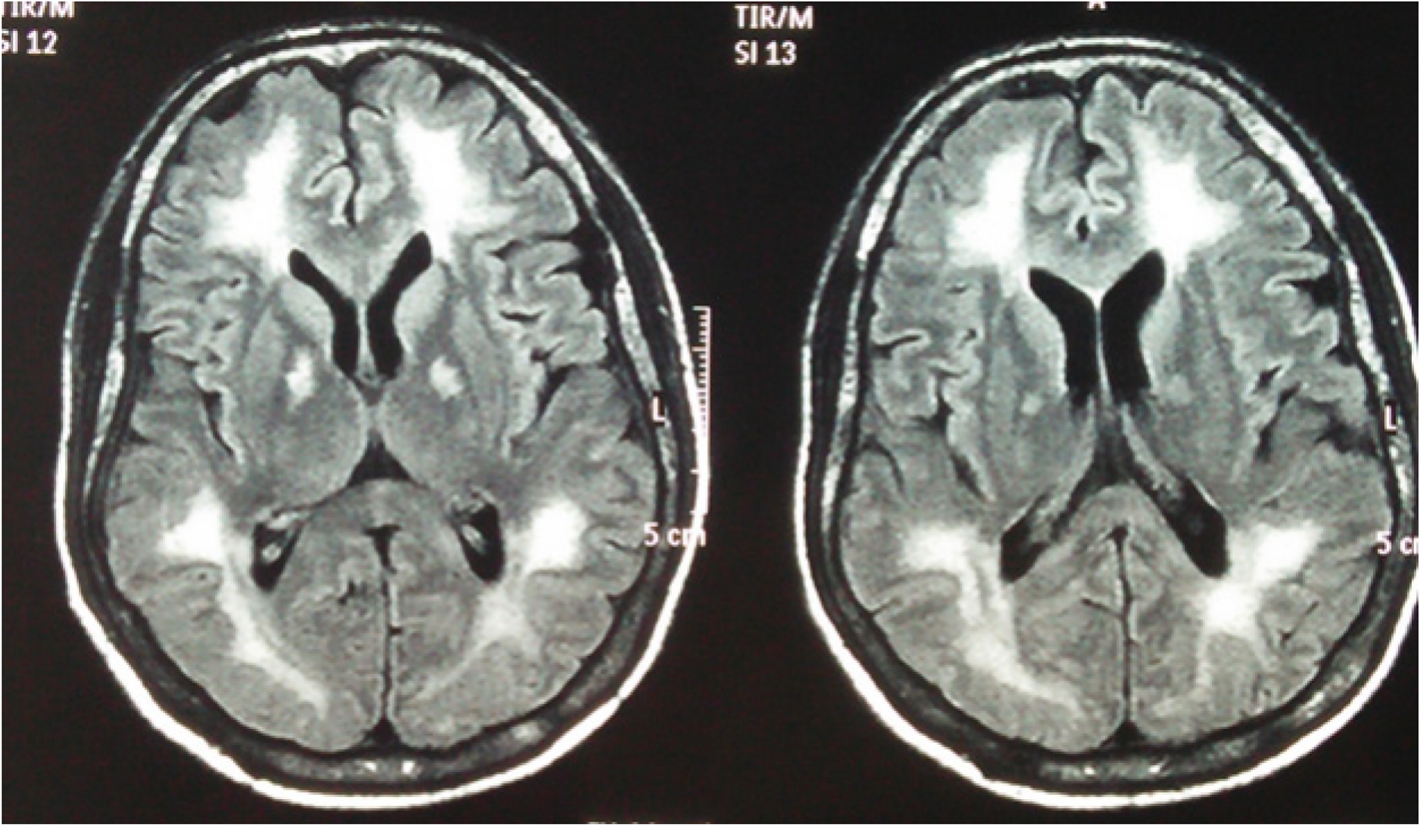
MRI brain shows diffuse high signal intensity involving subcortical white matter, globus pallidus on FLAIR images. Rest of the cerebral hemisphere and ventricular system were normal
